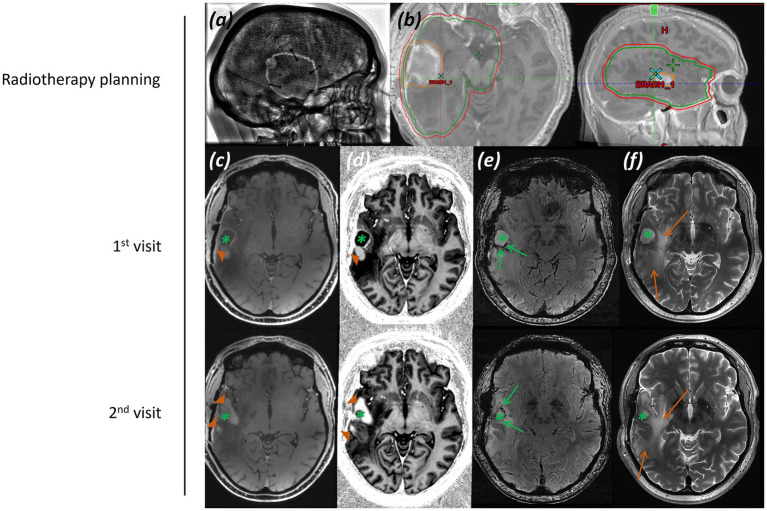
Patient 5: Glioblastoma, IDH-wildtype (CNS WHO grade 4), post-operative and post-radiotherapy. (a) CT image displaying craniotomy and titanium fixation plates, (b) radiotherapy planning maps in axial and sagittal planes, with gross tumor volume (GTV, orange), clinical target volume (CTV, green), and planning target volume (PTV, red) marked, and (c) second inversion time image from a T_1_-weighted MP2RAGE acquisition post-gadolinium contrast, (d) T_1_-weighted MP2RAGE UNI image post-gadolinium contrast, (e) SWI, and (f) T_2_-weighted TSE image, all at two time points 5 months apart, one month and six months post completion of radiotherapy. (Green asterisk* indicates resection cavity; green arrow indicates blood degradation products surrounding the resection cavity; orange arrowhead indicates post-contrast enhancement; orange arrow indicates abnormal white matter).
